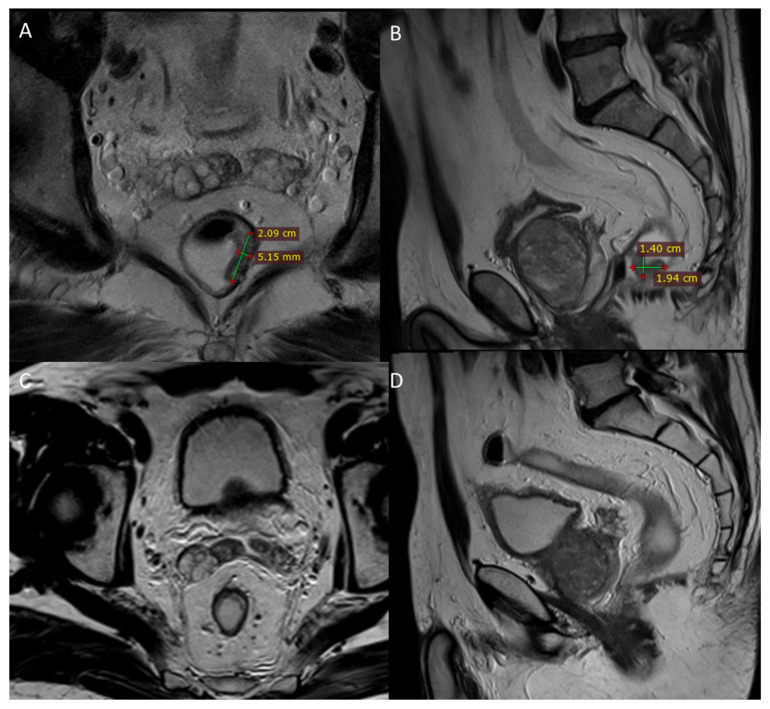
MR images (A,B) before treatment of second case and 2 months after ECT treatment (C,D).
